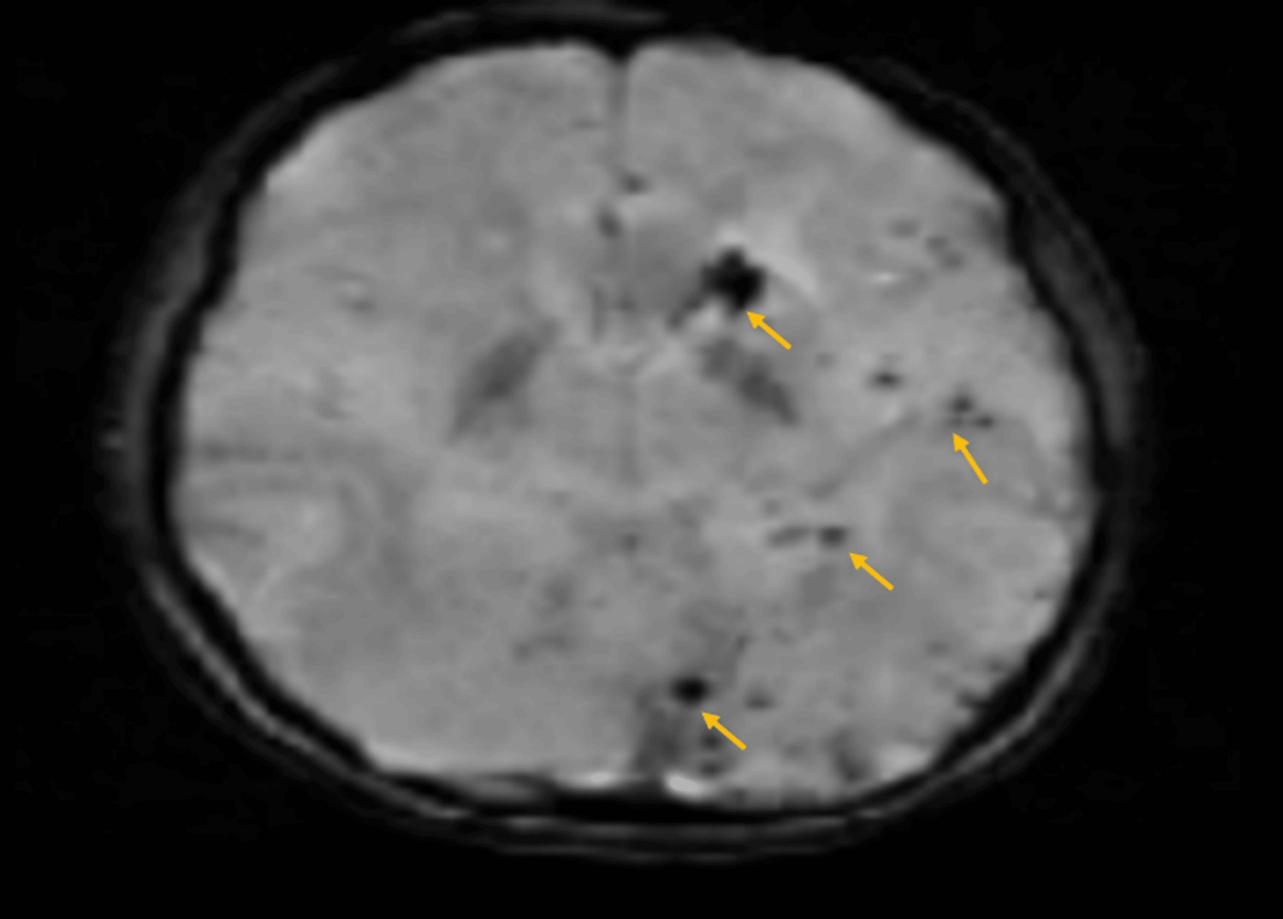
MRI (susceptibility-weighted imaging) of the brain showing tiny cystic spaces, multiple calcifications, and microhemorrhages involving the left gangliocapsular region, left parietooccipital, and left temporal cortex (yellow arrows).
